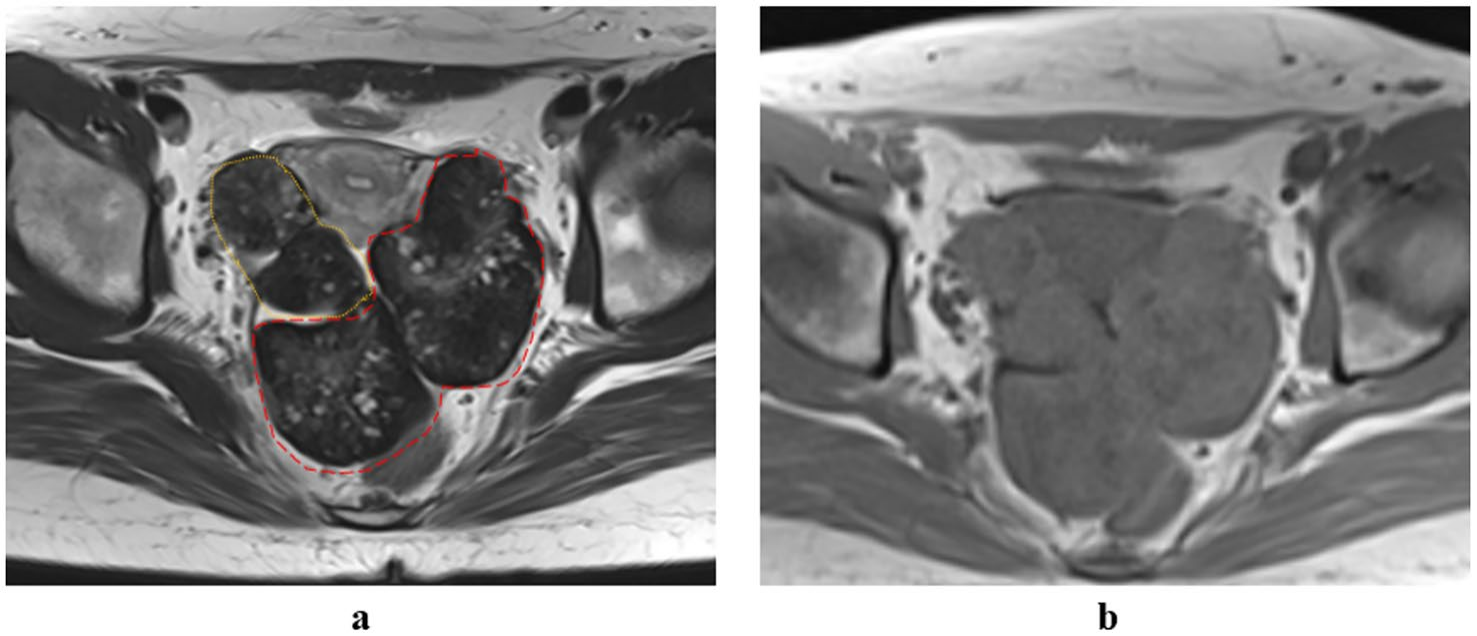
Magnetic resonance imaging findings of the present case. (a) T2‐weighted image, (b) T1‐weighted image. Solid masses were observed in both ovaries. The right (thin‐dotted line) and left (wide‐dotted line) ovaries contain small follicles and are surrounded by low signals on T2‐weighted imaging, suggesting stromal proliferation.
